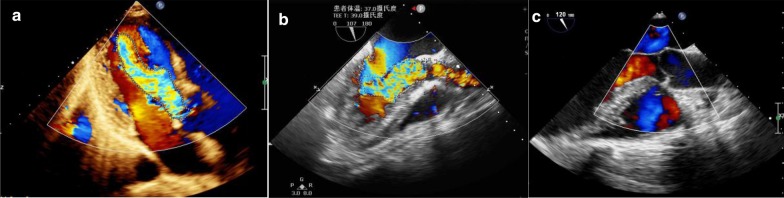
a Post-anaesthetic transthoracic echocardiography and b transoesophageal echocardiography showed severe aortic regurgitation. c Post-operative transoesophageal echocardiography showed no significant aortic regurgitation
